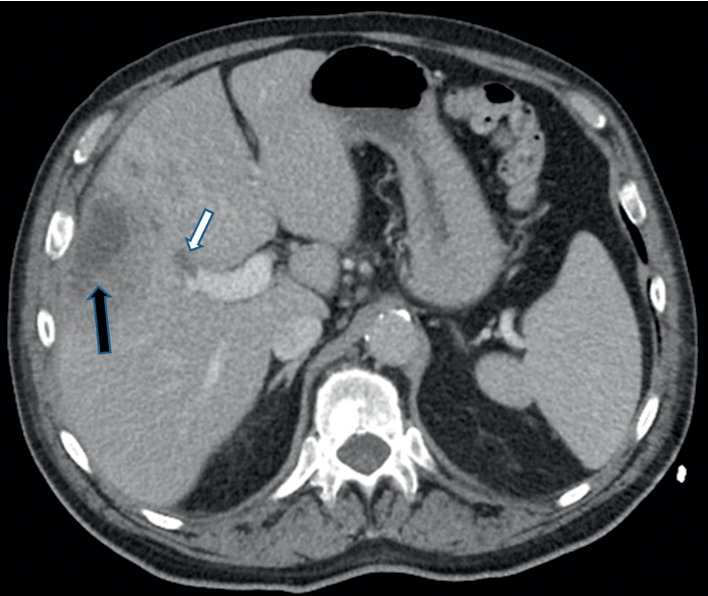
Contrast-enhanced axial CT image of the upper abdomen demonstrating the liver abscess (black arrow) and thrombus in the portal vein (white arrow).
